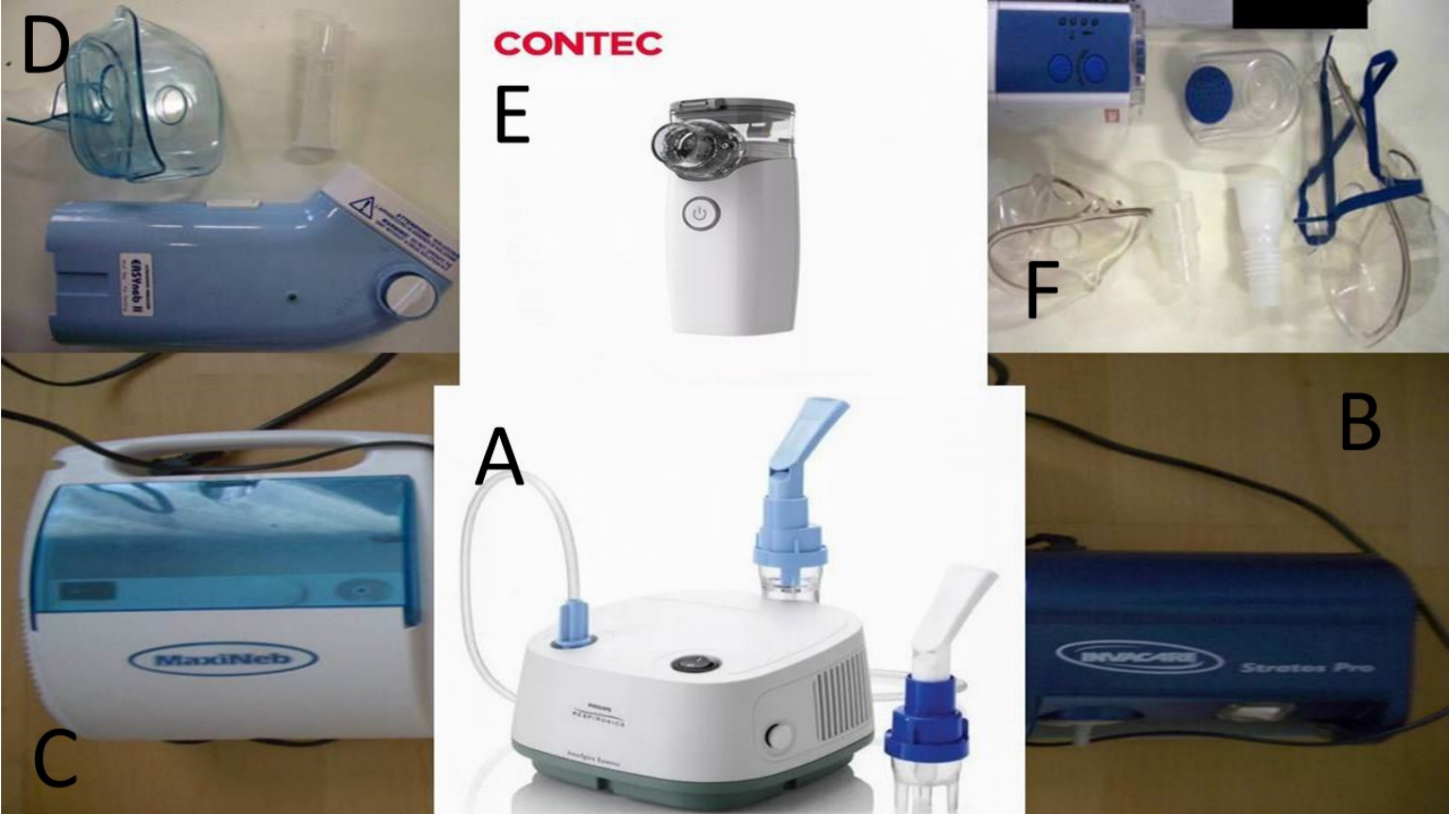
A) Philips jet-nebuliser, B) Invacare jet-nebuliser, C) Maxineb jet-nebuliser, D) Easybeb ultrasound nebuliser, E) Contec ultrasound nebuliser, F) Omron ultrasound nebulizer.
